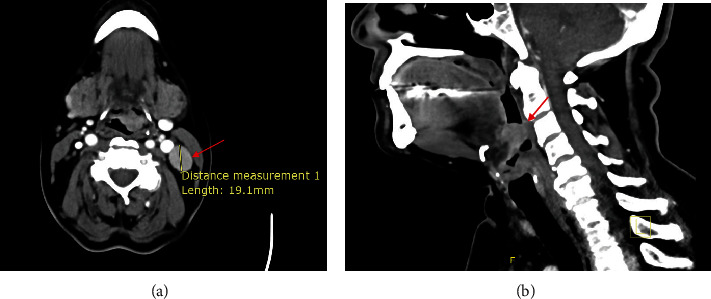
Computed tomography (CT) scan of the neck. (a) Axial view demonstrating left Level III cervical lymphadenopathy. (b) Sagittal view showing a lesion in the left supraglottic hemilarynx.
